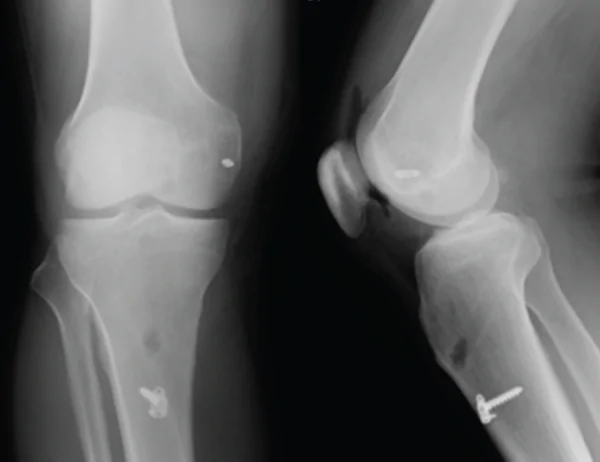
Anteroposterior and lateral radiographs of the right knee obtained immediately after surgery, showing the EndoButton positioned distal to the medial femoral epicondyle.
